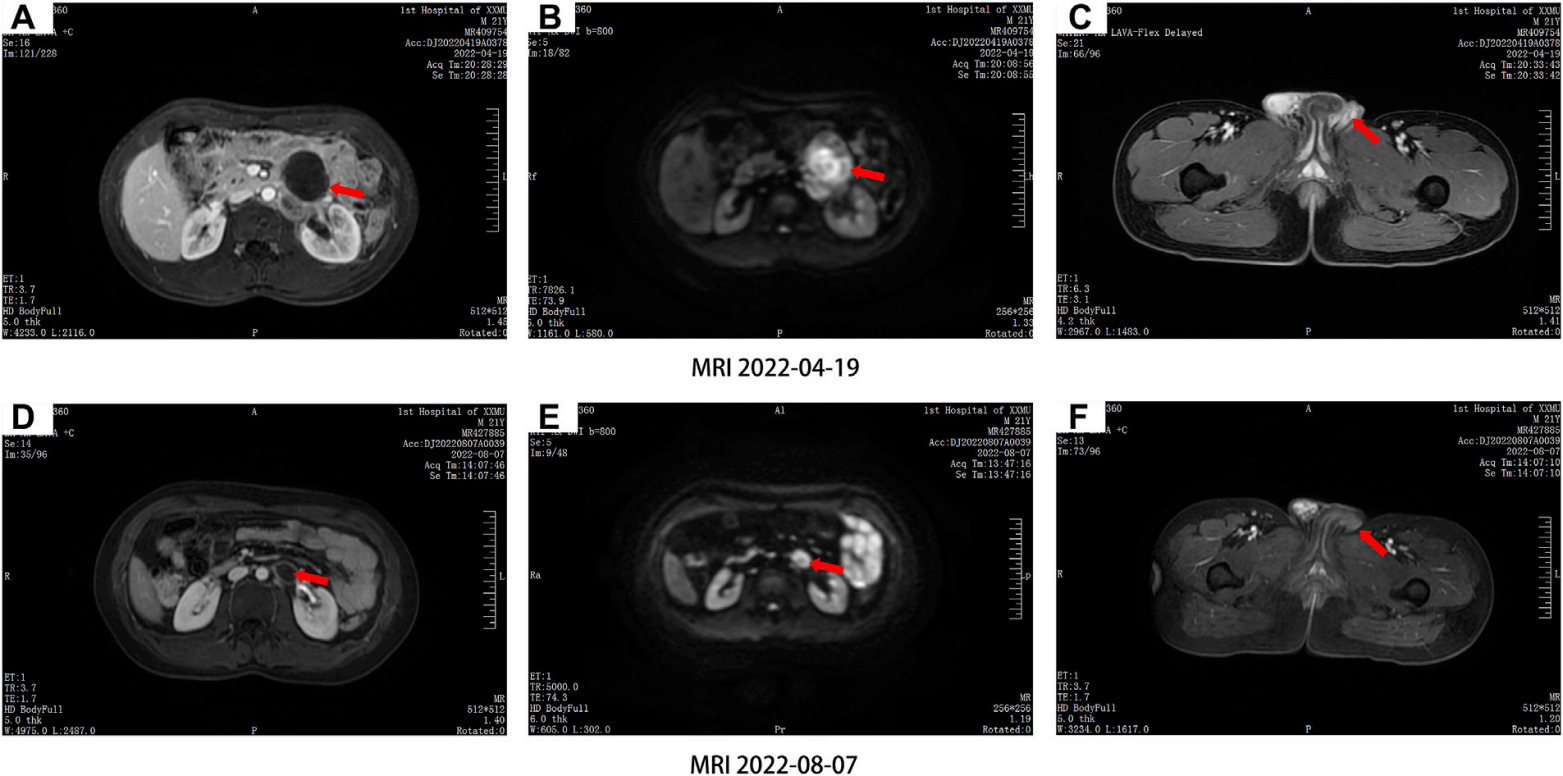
Comparison of abdominal magnetic resonance imaging (A,B,C) before and (D,E,F) after chemotherapy, the left retroperitoneal lymph nodes were significantly reduced.
